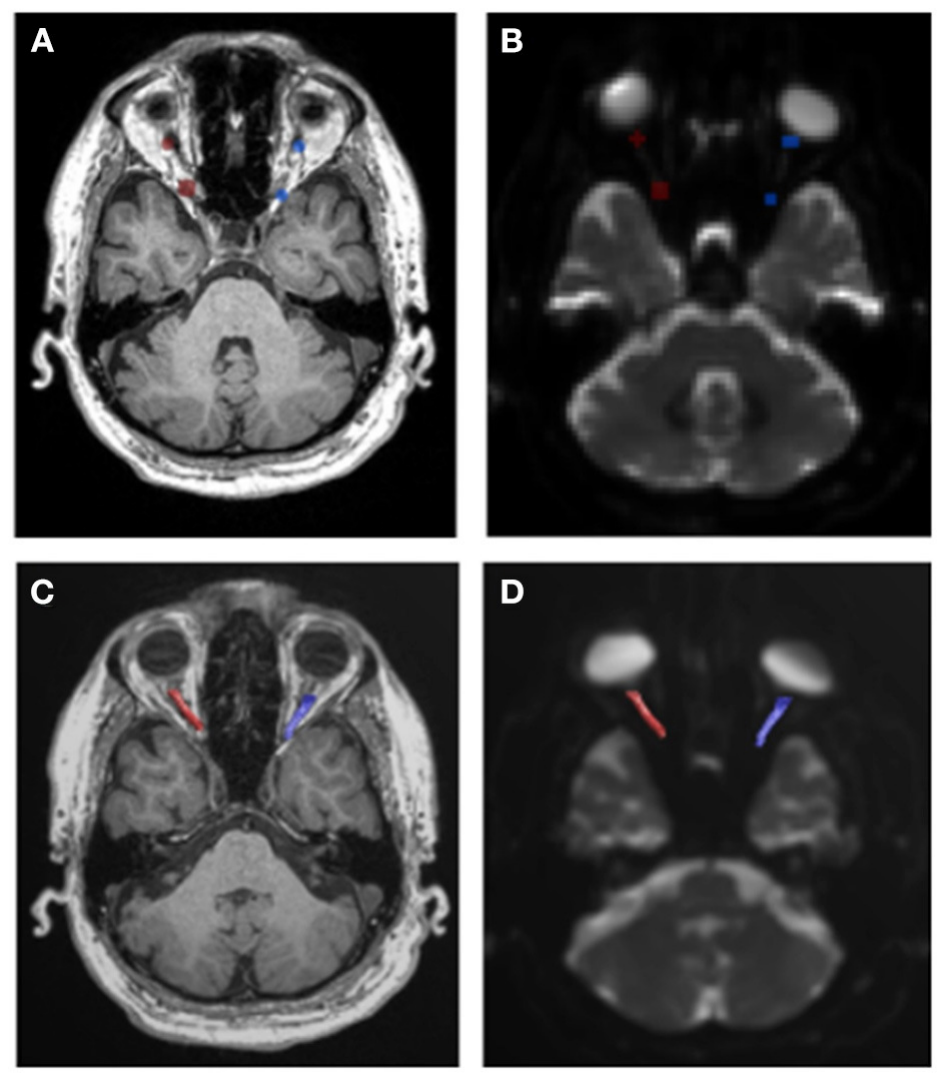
Geometric distortion in diffusion magnetic resonance images. (A,B) When there was a difference between the locations of the ROIs of the T1-weighted image and b0 image, we choose b0 images to locate the ROI. (C,D) Final tractography is well-matched with the b0 image rather than the T1-weighted image.
